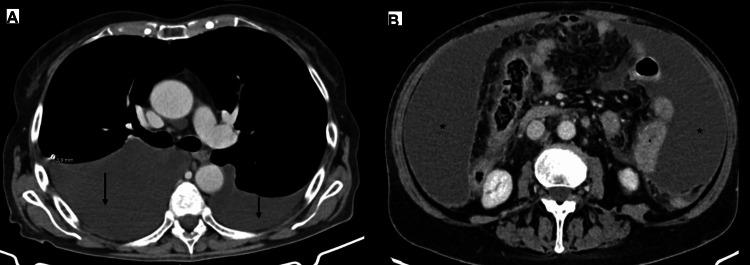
Body computed tomography (CT) findings. (A) Thoracic CT (left panel) showed bilateral pleural effusion (arrows) with passive atelectasis of lung parenchyma and a 3.9 mm calcified granuloma in the right upper lobe posterior segment. No evident adenopathies were registered. (B) Abdominal CT (right panel) revealed moderate volume ascites (*), with thickening and enhancement of peritoneal layers, suggesting inflammatory or infectious peritonitis.
